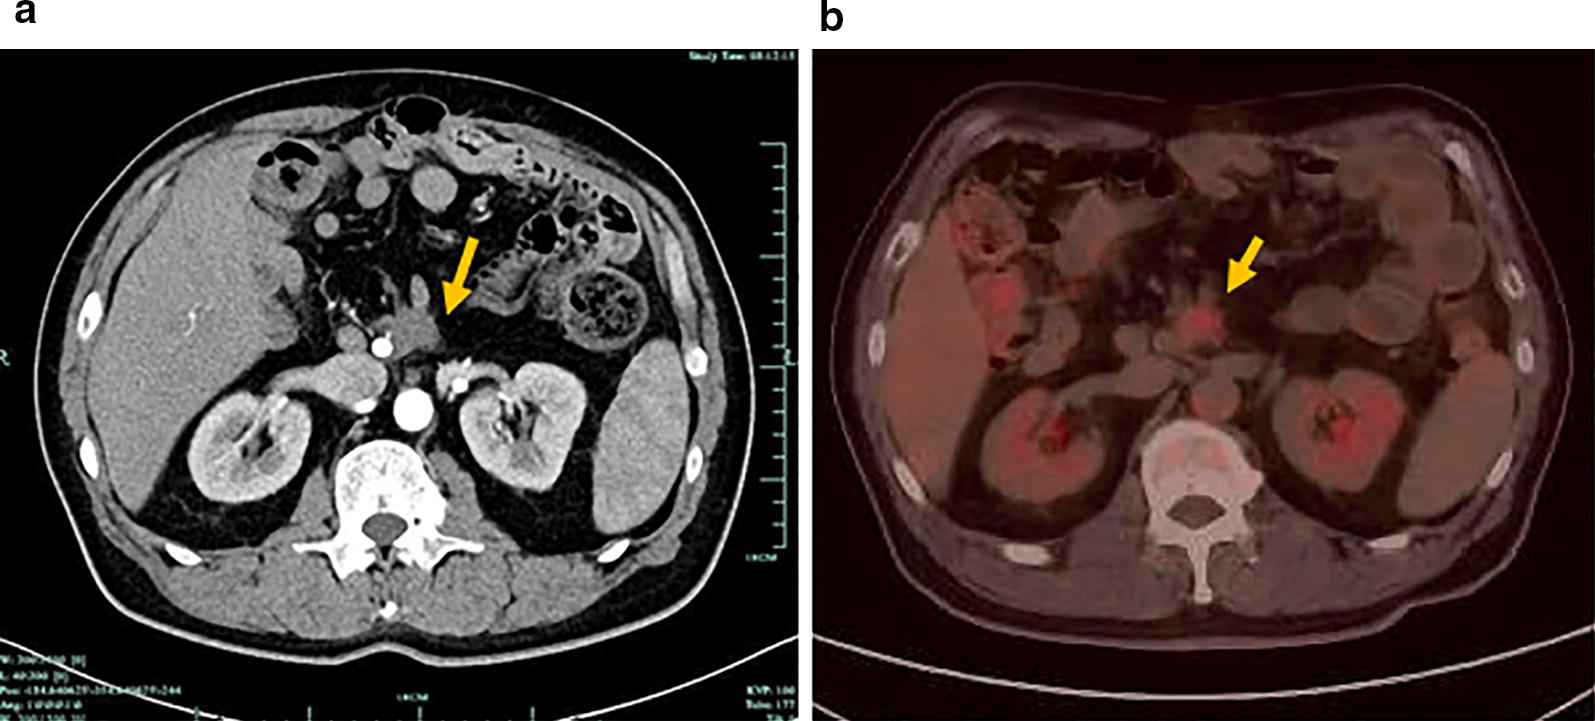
Computed tomography (CT) and PET/CT images of the metastatic lesion from a patient with duodenal adenocarcinoma. a CT scan shows the metastatic lesion (arrow) in the root of superior mesenteric artery and below transverse mesocolon. b PET/CT demonstrates malignant disease with FDG uptake (arrow) in the retroperitoneum
